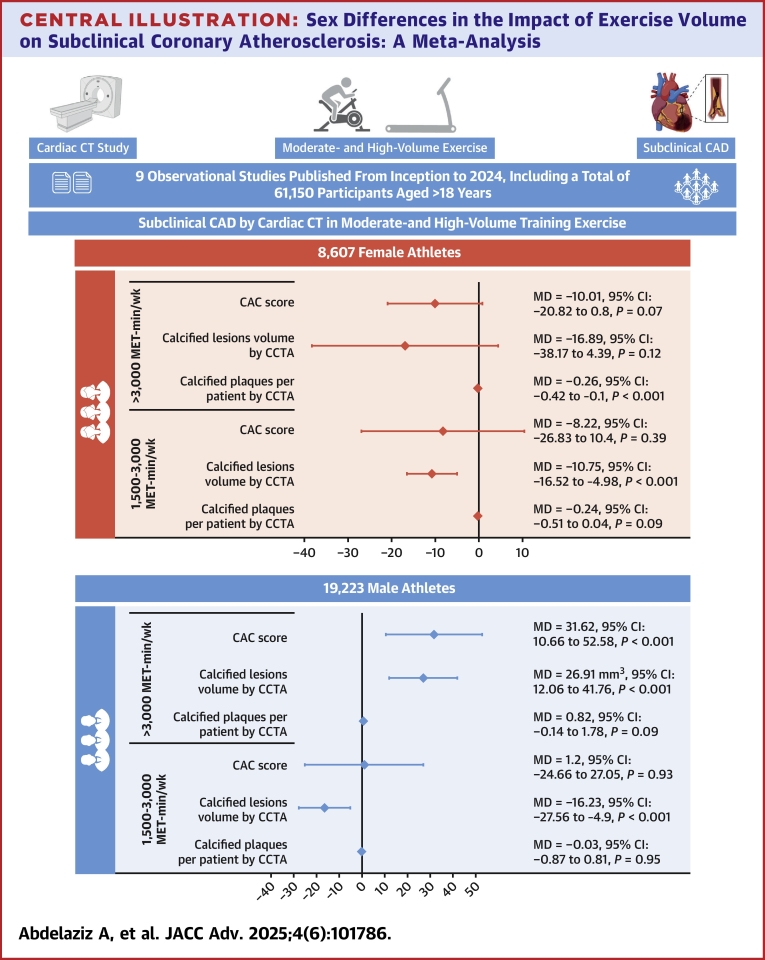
Sex Differences in the Impact of Exercise Volume on Subclinical Coronary Atherosclerosis: A Meta-Analysis CAD = coronary artery disease; CCTA = coronary computed tomography angiography; CT = computed tomography; other abbreviations as in Figures 2 and 4.
